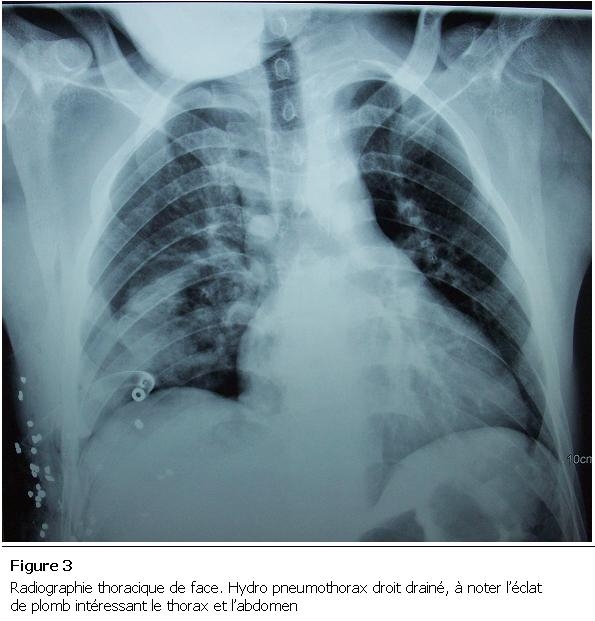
Radiographie thoracique de face. Hydro pneumothorax droit drainé, à noter l’éclat de plomb intéressant le thorax et l’abdomen
